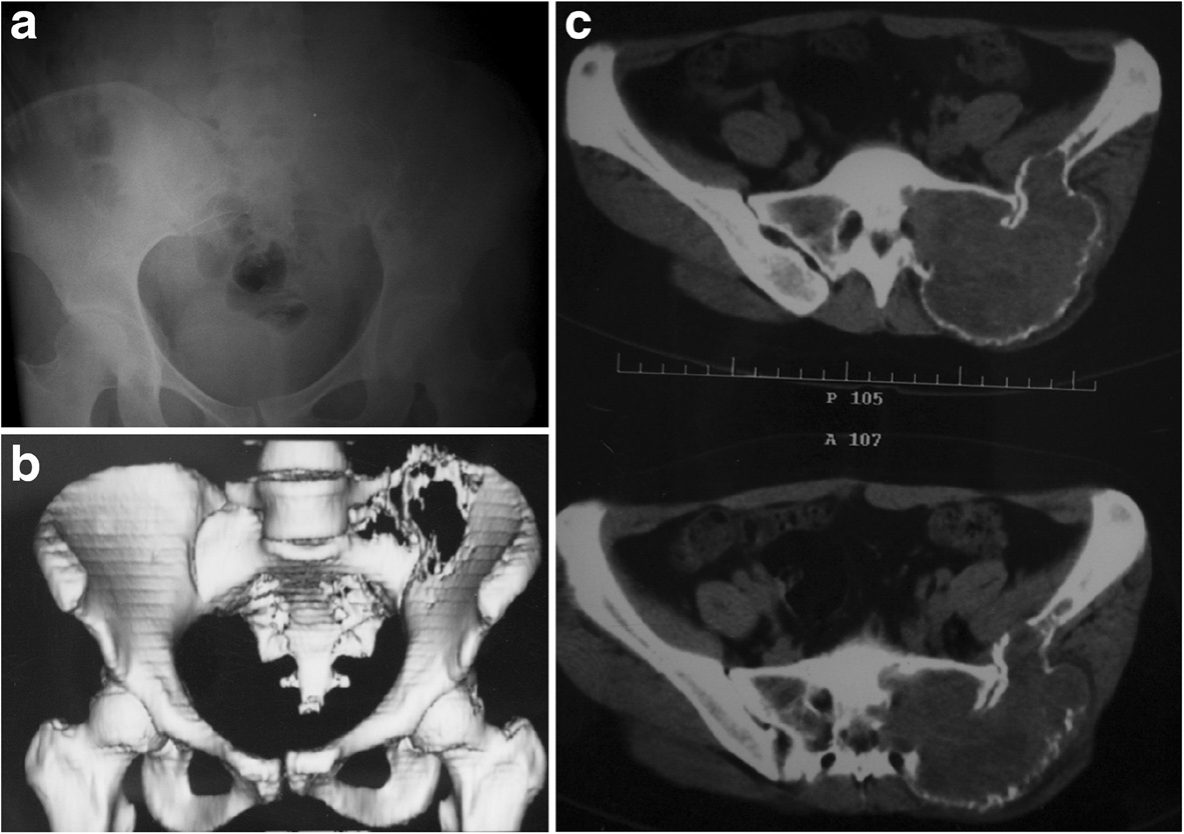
A 31-year-old woman presented with giant cell tumor involving left sacroiliac joint. Preoperative radiography (a) and computerized tomography (CT) (b) shows an eccentric, geographic, destructive, osteolytic lesion involving the sacrum and posterior superior iliac spine, with slight displacement of the pubic symphysis and left sacroiliac joint (c).
